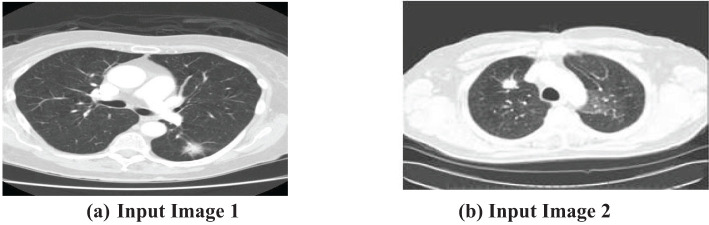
Input CT Lung Scan Images. (A) Input Image 1. (B) Input Image 2.
